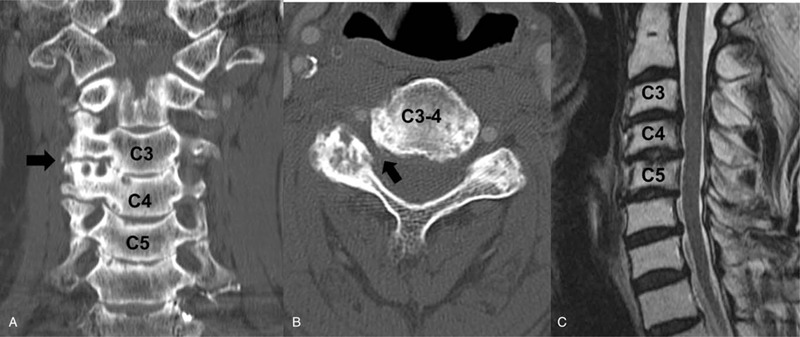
(A) The coronal image and (B) axial image on CT reveal severe foraminal stenosis of C3–4 and C4–5. (C) The sagittal image on MRI showing mild disc protrusion at C3–4–5. CT = computed tomography, MRI = magnetic resonance imaging.
